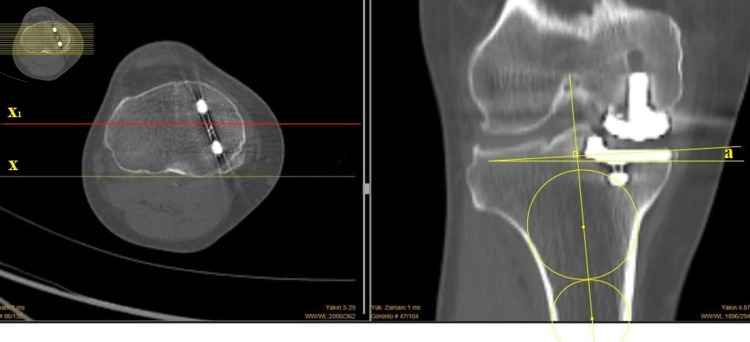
Coronal alignment on CT scans For coronal alignment on CT scans, the anatomical axis of the tibia was determined using two circles positioned 4-5 cm apart with the second circle placed as distally in the image as possible. The extended line connecting the two points at the center of the circles represented the longitudinal axis of the tibia. The coronal slices were set manually parallel to a line connecting the posterior tubercles of the tibia in the axial plane. At the level where the tibia was at its widest, coronal measurement of the implant was performed with the circle method, similar to the measurement performed on X-rays. CT: computed tomography
